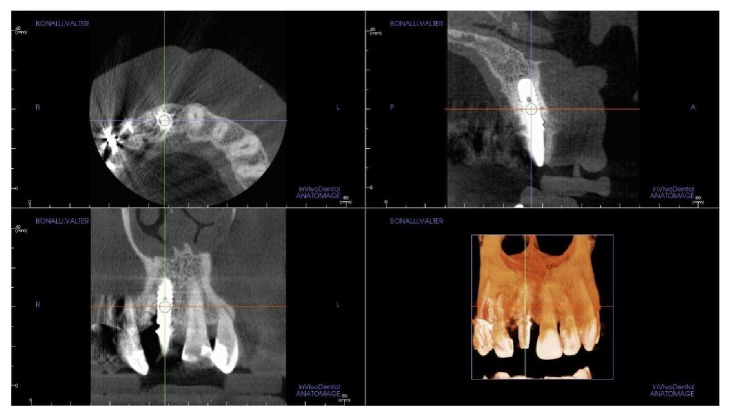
A third small FOV CBCT with 3D reconstruction confirmed excellent osseointegration of the implant with unchanged peri-implant marginal bone levels, indicating that the treatment proposed was able to restore the functional and aesthetic parameters.
